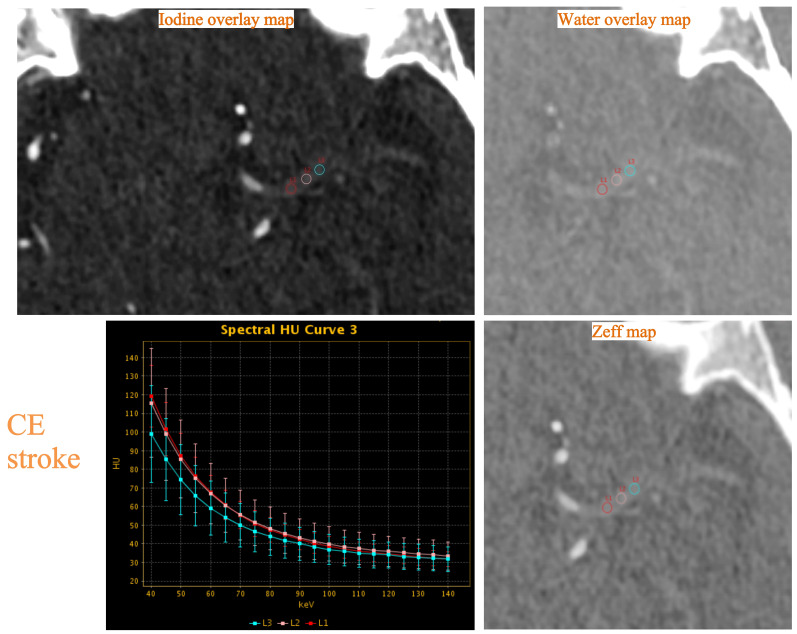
Example of thrombus dual-energy CT (DECT) variables assessment
